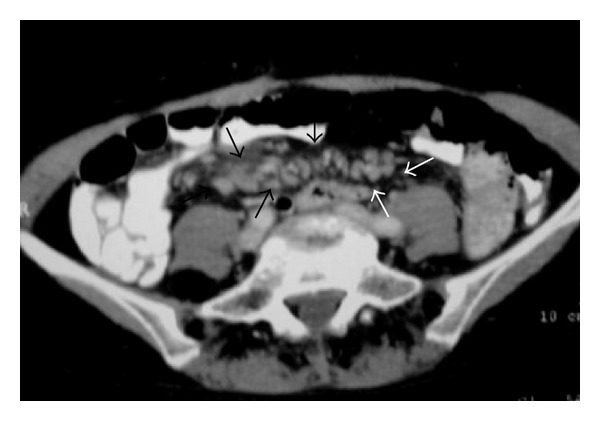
NHL in a 53-year-old woman. Axial pelvic contrast-enhanced CT shows mesenteric lymph nodes (arrows).
